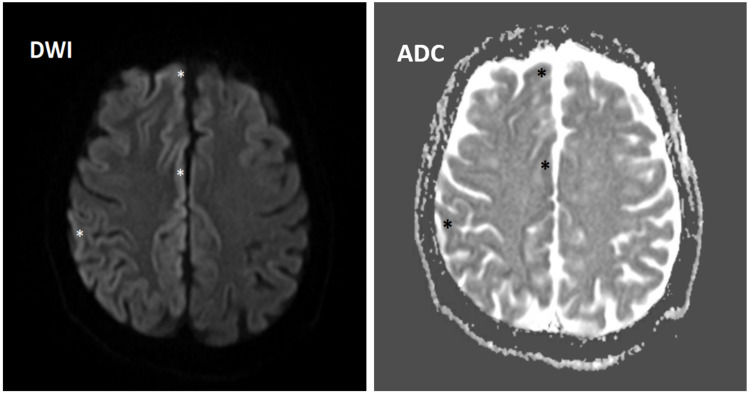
MRI of the brain showing diffusion restriction areas within the cortical sulci of the right frontal and parietal lobes (asterisks) in both DWI and ADC sequences. MRI = magnetic resonance imaging; DWI = diffusion-weighted images; ADC = apparent diffusion coefficient
